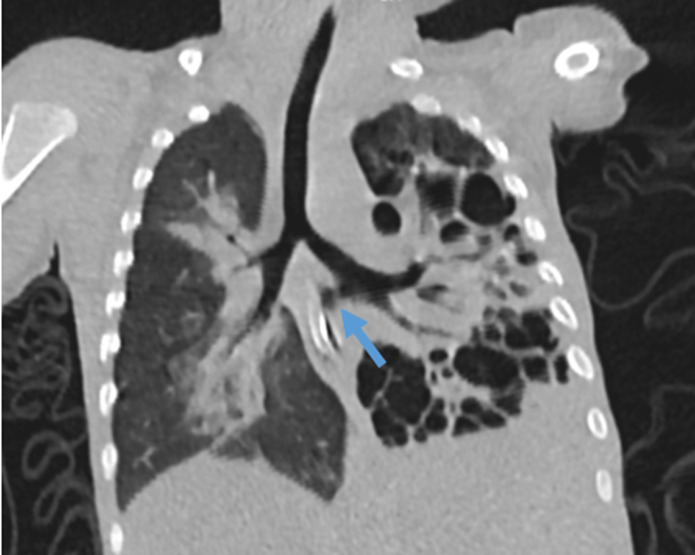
chest computed tomography coronal reformatted view showing a fistulous connection between a distal left main bronchus and the esophagus (arrow) and cystic changes
